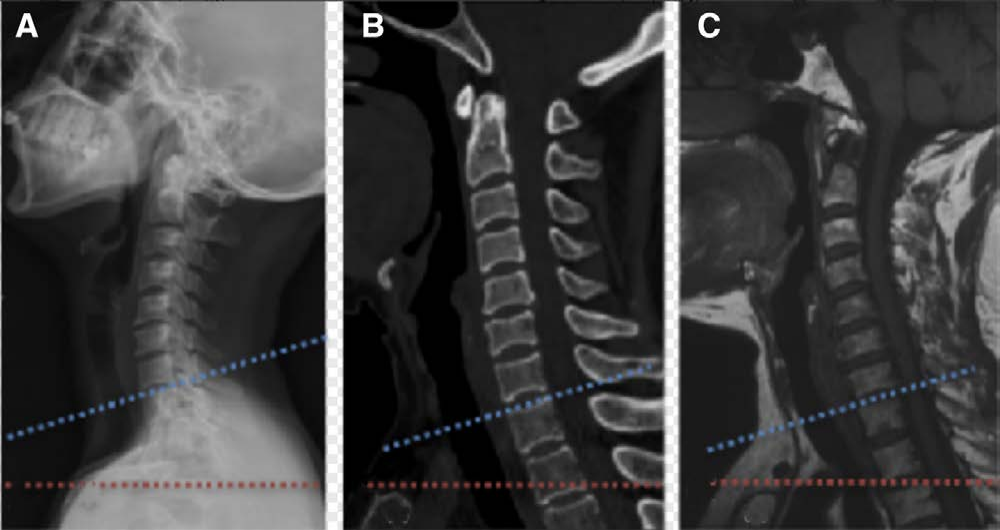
Cervical vertebra lateral radiography and MRI showed sternal notch is at the same level with C7/T1 intervertebral disc vertically.
